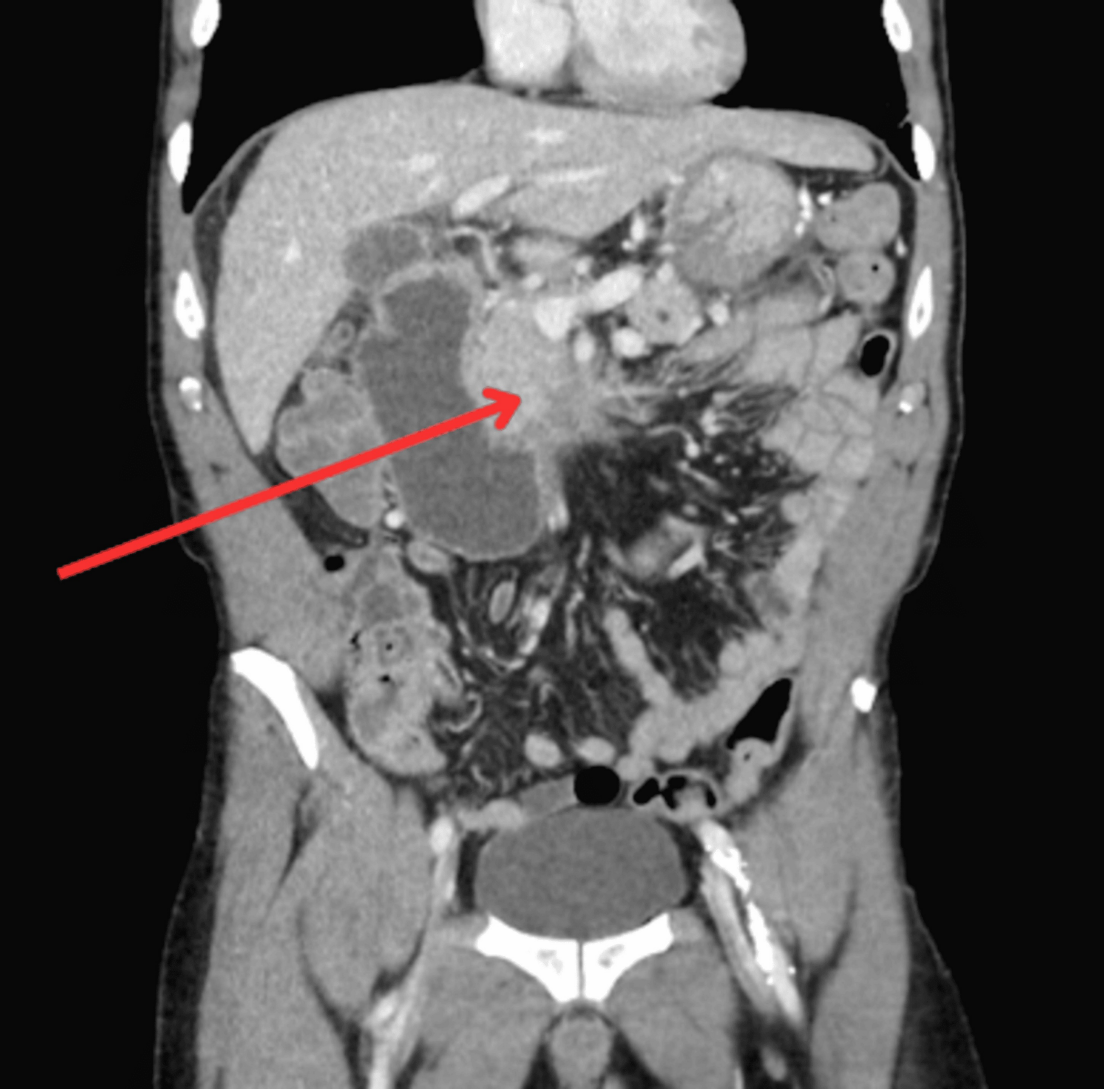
CT scan of the abdomen showing the fullness in the pancreas head with dilatation of the duodenum CT: computed tomography
